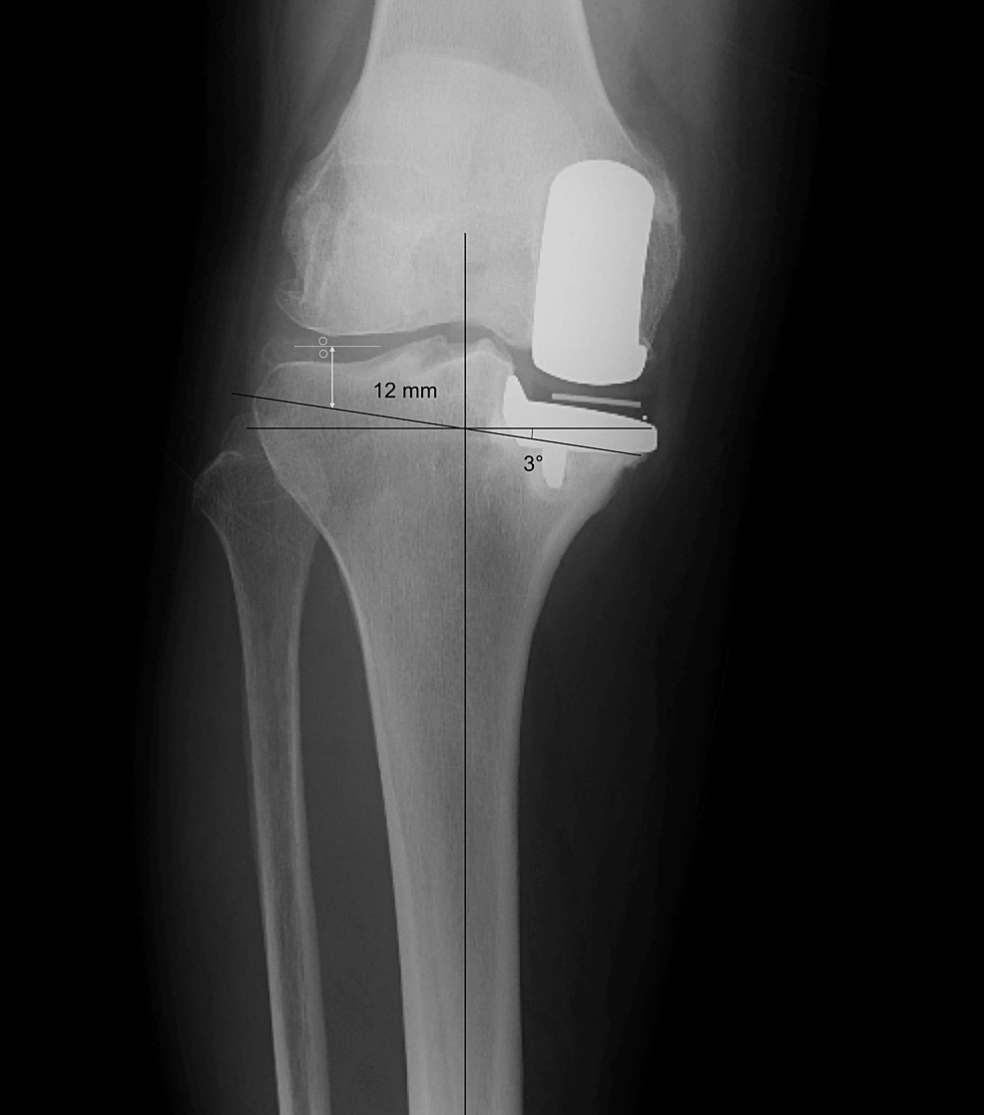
Preoperative radiographic evaluation. The estimated cutting line is drawn from the highest point of the intact medial tibial cortex with 3° varus forming a perpendicular line of the tibial axis. This line should be <12 mm from the lateral joint line.
